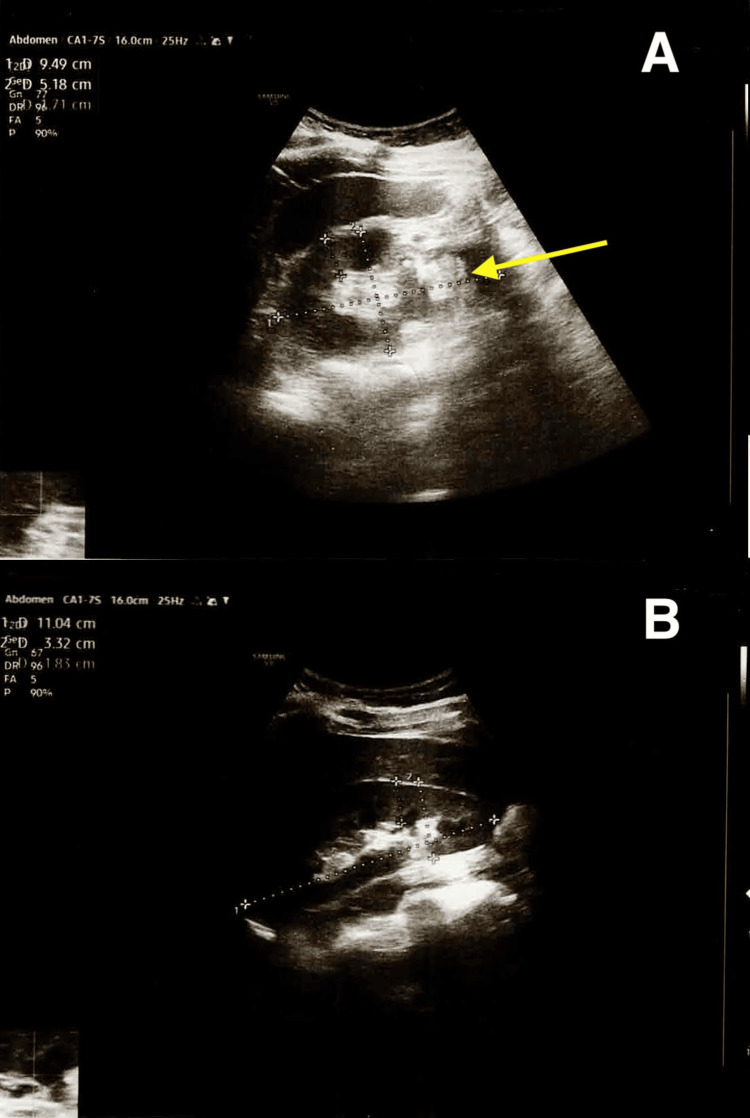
Ultrasonography of the right and left kidneys A: left kidney shows scarring of the lower half (yellow arrow) with increased parenchymal echogenicity; B: right kidney shows mildly increased parenchymal echogenicity with preserved corticomedullary differentiation.
